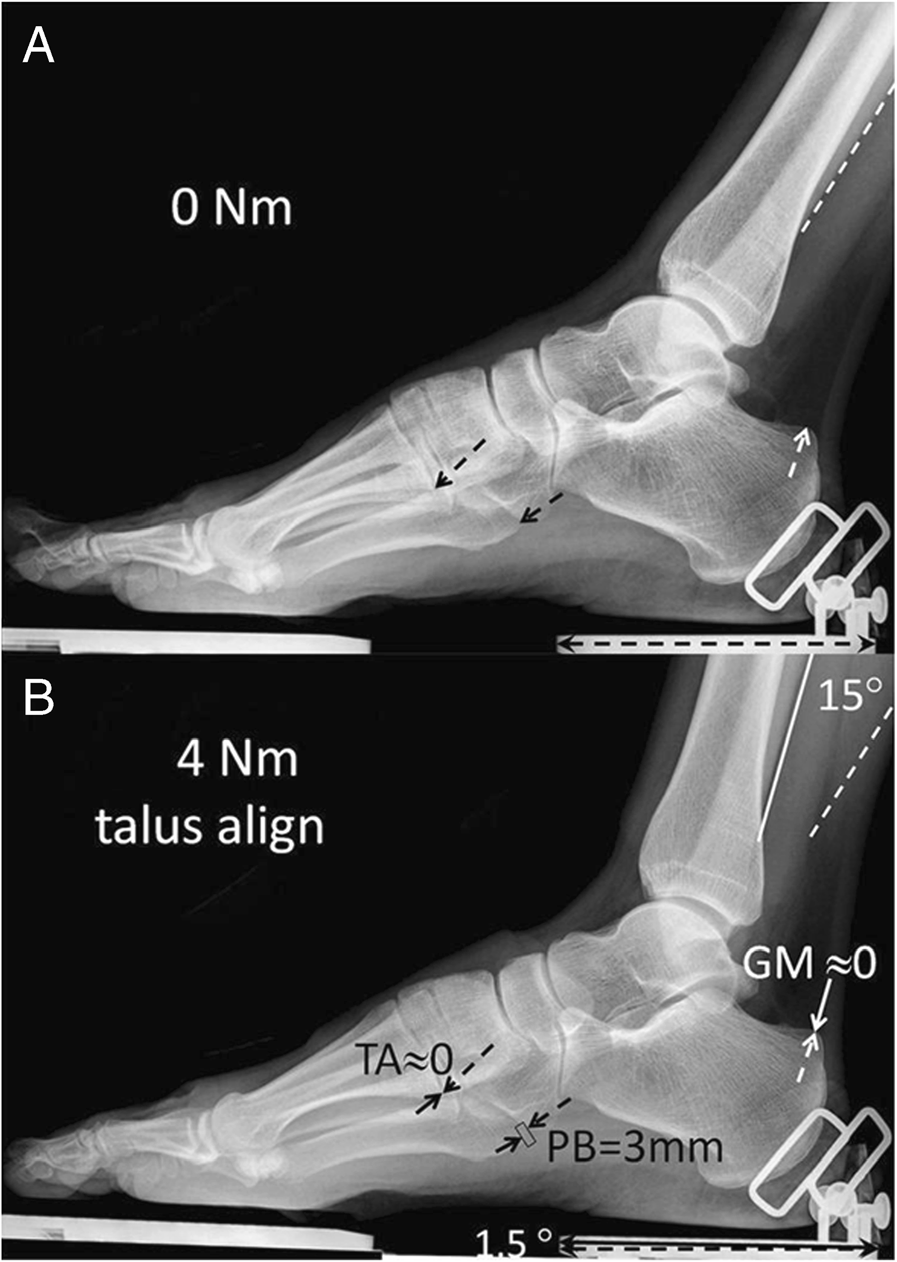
Analysis of X-rays for a TD adult subject after talus alignment. A. The foot in a position similar to that when exerting 0 Nm. Under high magnification markers (dashed lines and arrows) were placed for alignment and at particular insertion points of muscles and along the fibula. B. The foot in a position similar to that when exerting 4 Nm dorsal flexion. The talar bone was aligned with that in A. Such talar bone alignment allows distinction of contributions to foot plate movement and muscular length changes by foot deformation and crural bone movements respectively. The markers of A. were copied to image B. (dashed lines or arrows). New markers (solid lines or arrows) placed on the reference points in the new condition. Length changes were estimated (rectangles) for GM (m. triceps surae). TA (m. tibialis anterior) and PB (m. peroneus brevis) and angular changes for the fibula and foot plate, as well as calcaneal angle (not shown). The arrow on the heel part of the foot plate is used for calibration (actual dimension = 8.98 cm).
